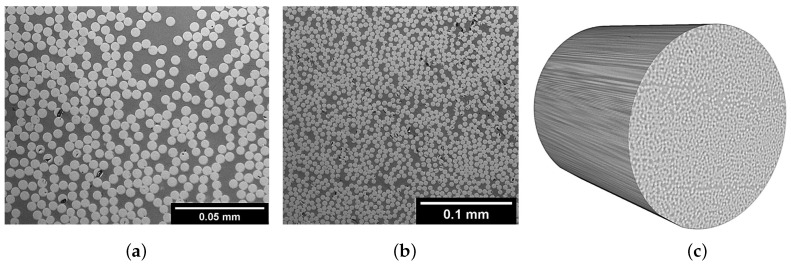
Inspection of IM7/PEEK; (a) A high magnification. (b) A low magnification. (c) 3D CT image.
